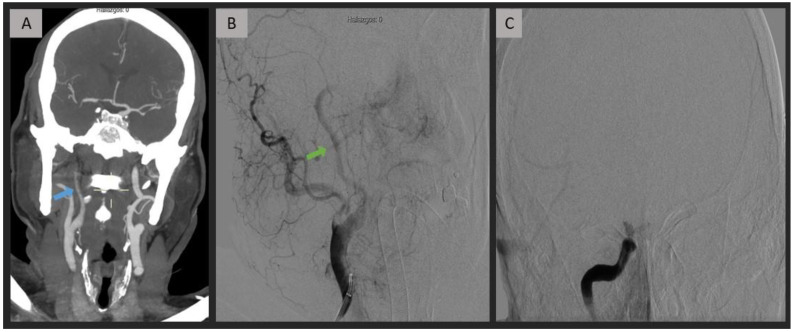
Acute intracranial ICA occlusion caused by thromboembolism. CTA (A) demonstrates a progressive contrast decay in the extracranial region, extending towards the distal right ICA (blue arrow), while maintaining patency of the terminal bifurcation. DSA images (B,C) confirm an isolated intracranial occlusion while indicating the patency of the extracranial segment (green arrow). ICA = internal carotid artery; CTA = computed tomography angiography; DSA = digital subtraction angiography.
